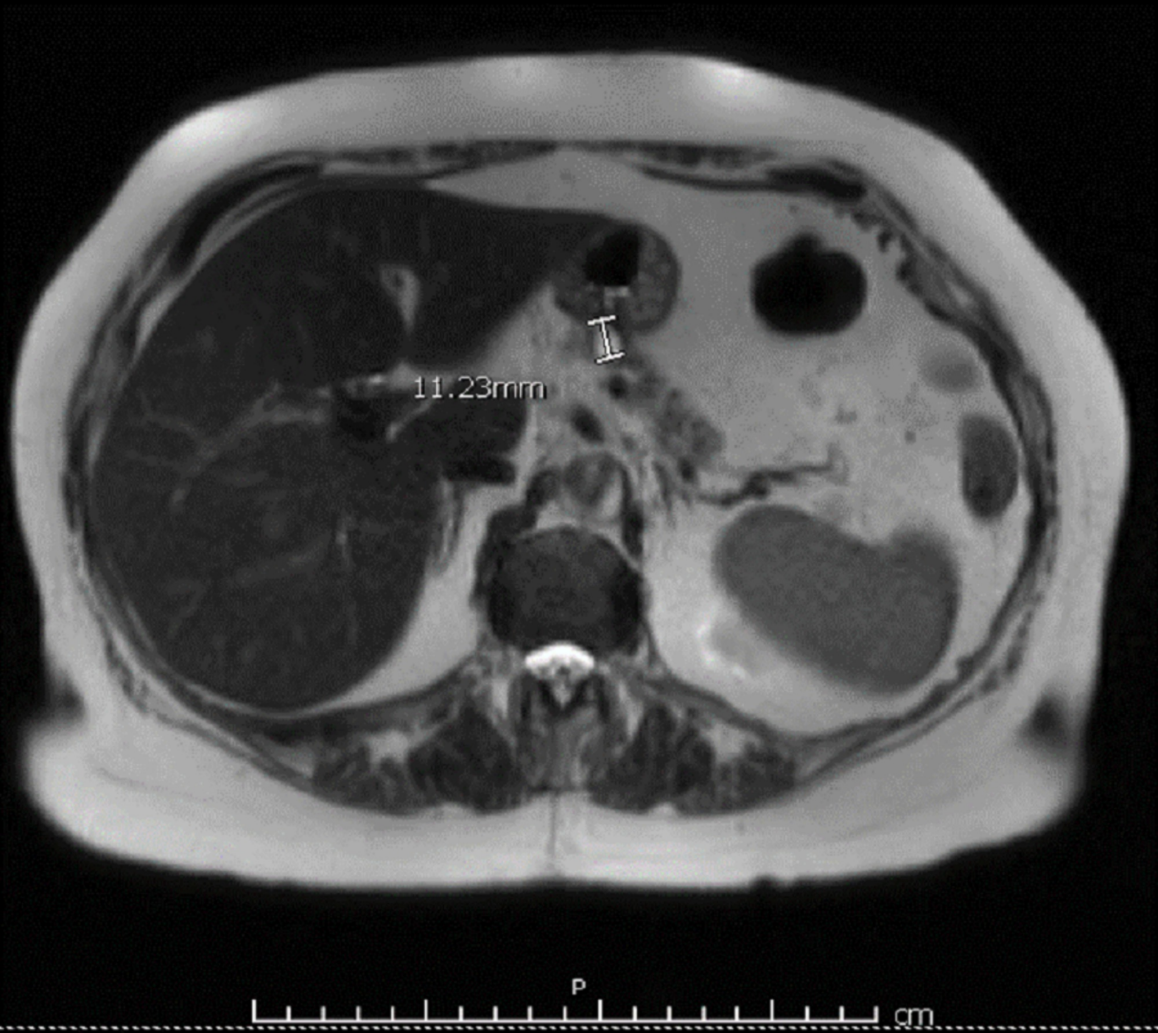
Abdominal MRI with and without contrast showing a 1.1-cm cystic lesion in the body of the pancreas without internal enhancement or septations.
